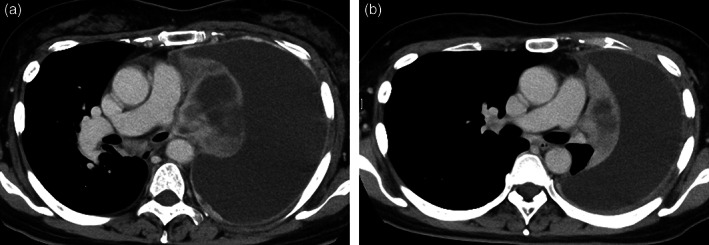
Tepotinib was administered with a reduction in tumor size and decrease in pleural effusion. (a) Chest computed tomography (CT) immediately prior to starting tepotinib. (b) Chest CT performed 43 days after the initiation of tepotinib showing shrinkage in tumor size and decrease in pleural effusion
